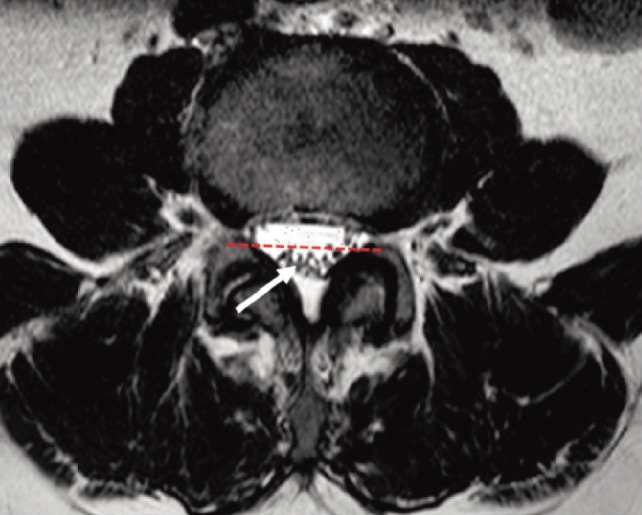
MRI T2 image of a patient with negative nerve root sedimentation sign. A negative sign was when the majority of the lumbar nerve root (arrow) was located in the dorsal half (dotted line) of the dural sac.
